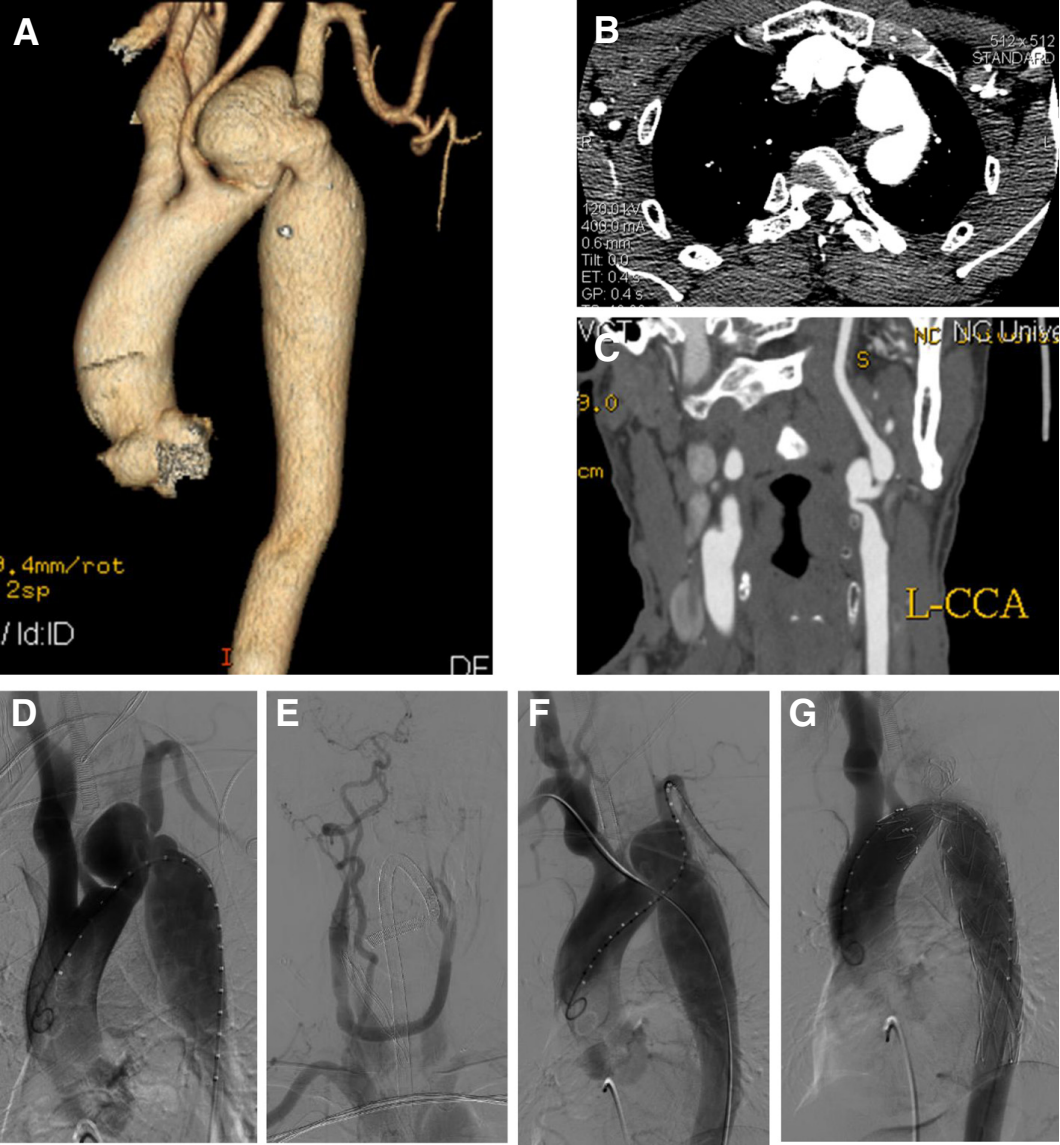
CTA demonstrated an aortic arch with 50 mm sized saccular aneurysm at 3 mm distal to the LCCA, aortic arch coarctation in the size of 12 mm (A, B), and a LICA aneurysm (C). DSA was in agreement with the CTA images (D) and showed patency of the carotid to carotid bypass (E); Brachial-femoral stretch guidewire technique was performed (F); The completion aortogram revealed no evidence of endoleak; disappearance of the aortic arch aneurysm lumen (G).
